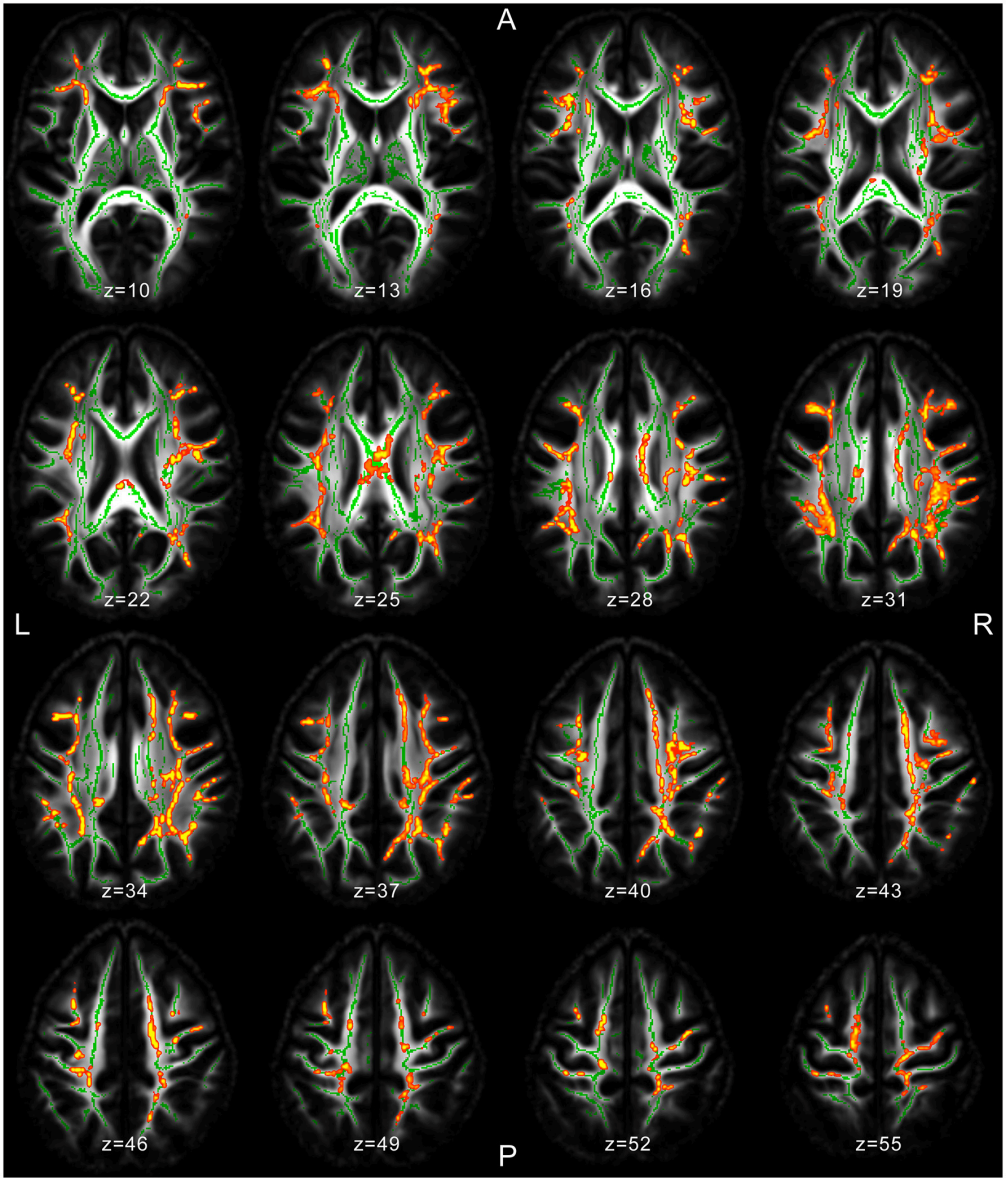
MRI images of significant TBSS clusters in patients with JME compared to healthy controls superimposed to the skeleton (light green).
